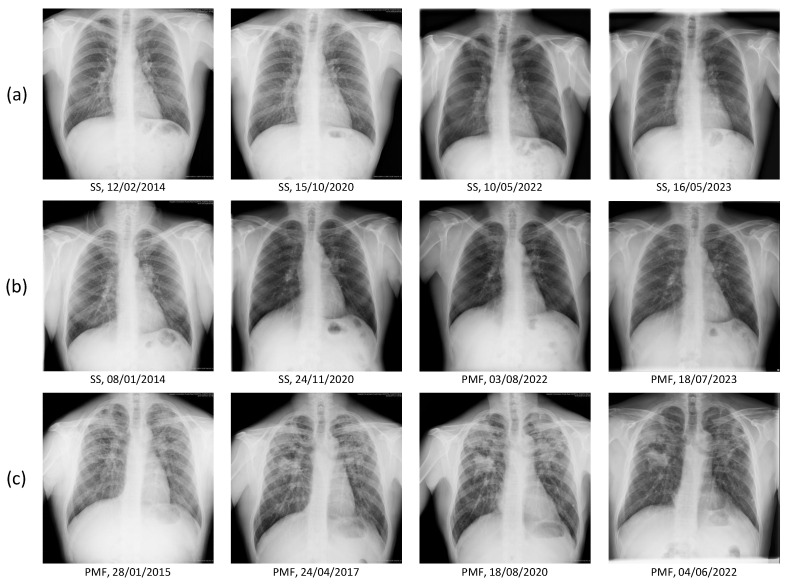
Representative longitudinal chest radiographs from three study participants. Each row corresponds to a single patient. (a) Patient initially diagnosed with simple silicosis (SS) and showing stable radiological findings over several follow-up dates. (b) Patient with progressive disease course, evolving from SS to progressive massive fibrosis (PMF) during follow-up. (c) Patient diagnosed with PMF at baseline and monitored across multiple time points.
